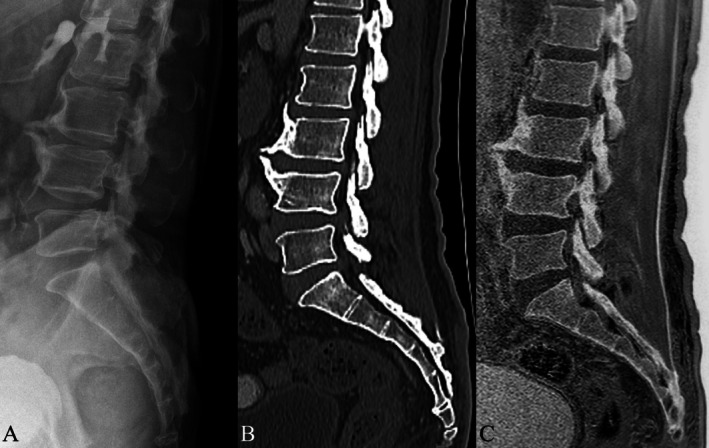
Lateral lumbar radiograph (A) with sagittal reformatted CT (bone window) (B) and FRACTURE MRI (C) demonstrating anterior L3 and L4 osteophytes.
